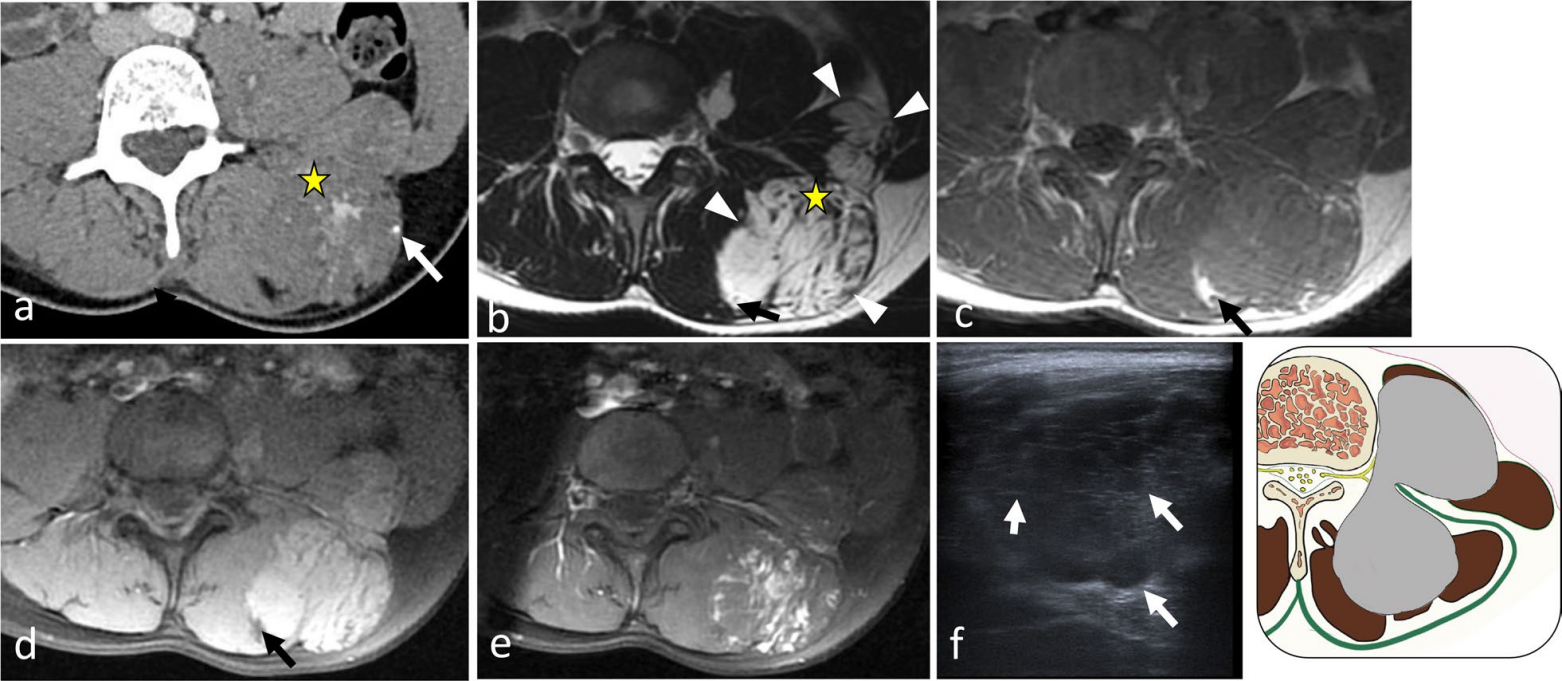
Haemangioma* in a 37-year-old female presenting with mild paraspinal pain and soft and slow-growing tumefaction. Contrast-enhanced axial CT scan reconstruction (a), axial T2WI (b) and axial T1WI (d) show a bilobed mass in the erector spinae muscles, the psoas muscle and the quadratus lumborum muscle with phlebolite (white arrow) and fat content (black arrow). Contrast-enhanced axial T1WI with fat saturation (e) demonstrates heterogeneous enhancement (a). On US, the mass appears as an ill-defined hypoechoic mass with multiple spaces within (white arrows) (f). The yellow star shows the epicentre of the mass
